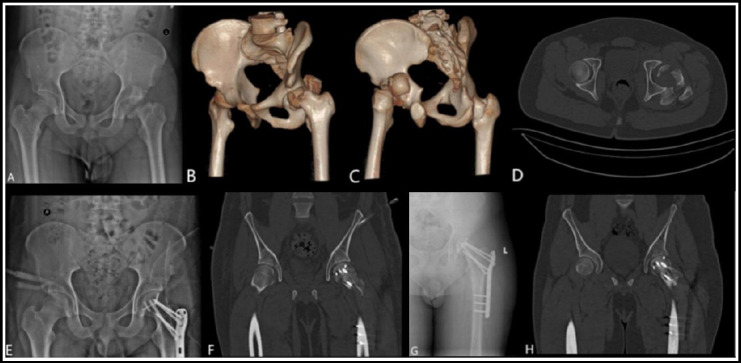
A nineteen-year-old male with left Pipkin Type-III fracture. Preoperative X-ray. (B-D) Preoperative CT showing comminuted fractures. (E) Postoperative X-ray. (F) Postoperative CT showing good reduction. (G) Two-year follow-up X-ray. (H) Two-year follow-up CT showing no necrosis and good healing.
